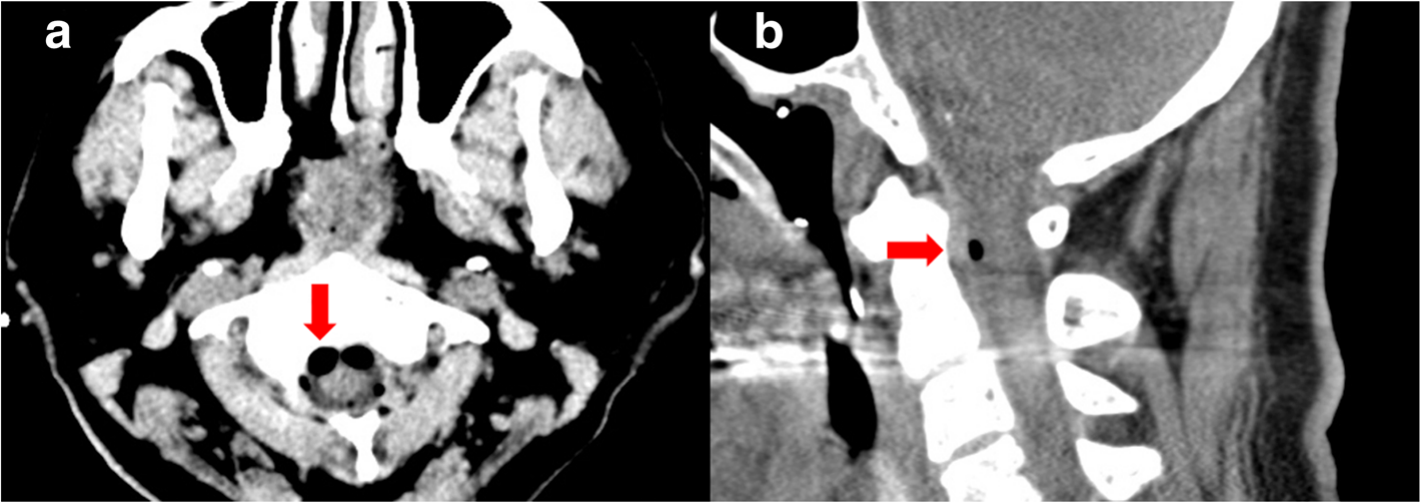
Brain scan showing air (red arrow) in the spinal canal and prepontine cistern (a). Spinal computed tomographic scan showing intradural free air (red arrow) at the C3 level (b)
